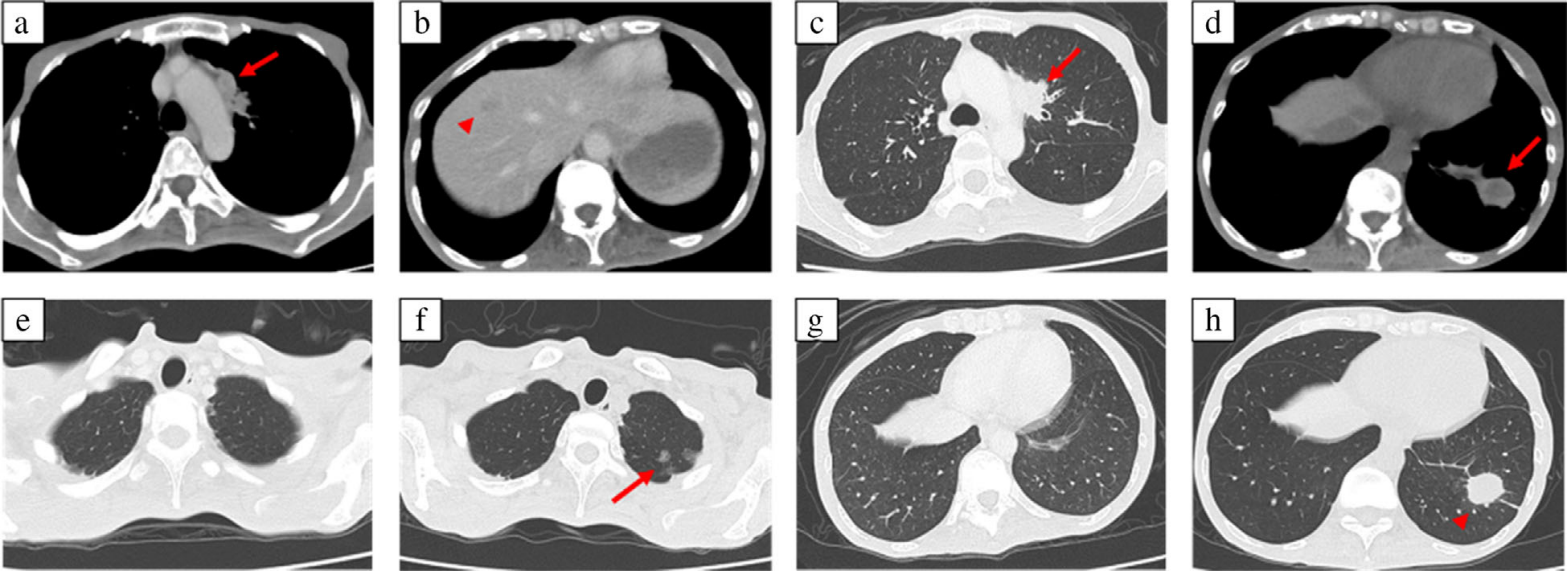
Whole‐body computed tomography (CT) scans. (a, b) The mediastinal window shows lymph node metastasis (arrow) and hepatic metastasis (arrowhead) before treatment. (c) The parenchymal window shows lung tumor before treatment (arrow). (d) The mediastinal window shows a low‐density area mimicking lung abscess or tumor recurrence on day 101. (arrow). (f, h) The parenchymal window shows ground glass opacity (arrow) and a nodule (arrowhead), which indicate pneumonitis caused by osimertinib on day 101, compared to (e, g) chest CT before treatment.
